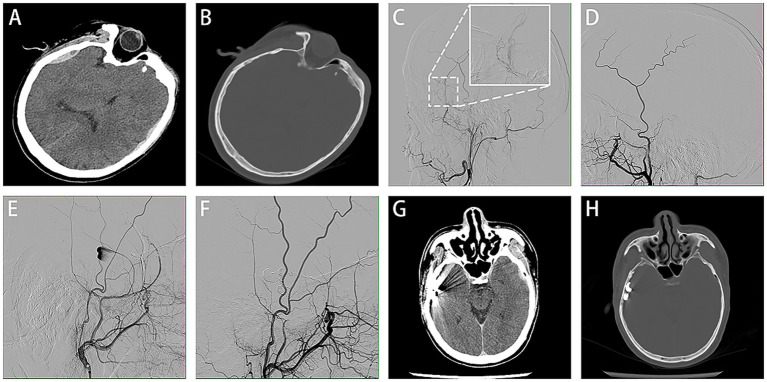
Case 3 representative images. Initial CT shows a left frontotemporal subdural hematoma and a right temporal EDH with an internal hypodense nodule (A) and an associated temporal bone fracture on bone window imaging (B). Selective left ECA angiography revealed abnormal vascular dilatation with active contrast extravasation within the region outlined by the white dashed box. This area is shown at higher magnification in the white solid box (C). The lesion was embolized subsequently (D). Selective right ECA angiography showed a heart-shaped pseudoaneurysm originating from the posterior branch of the MMA (E). The MMA was embolized promptly (F). Follow-up CT confirmed that the size of the hematoma was not increased (G,H).
